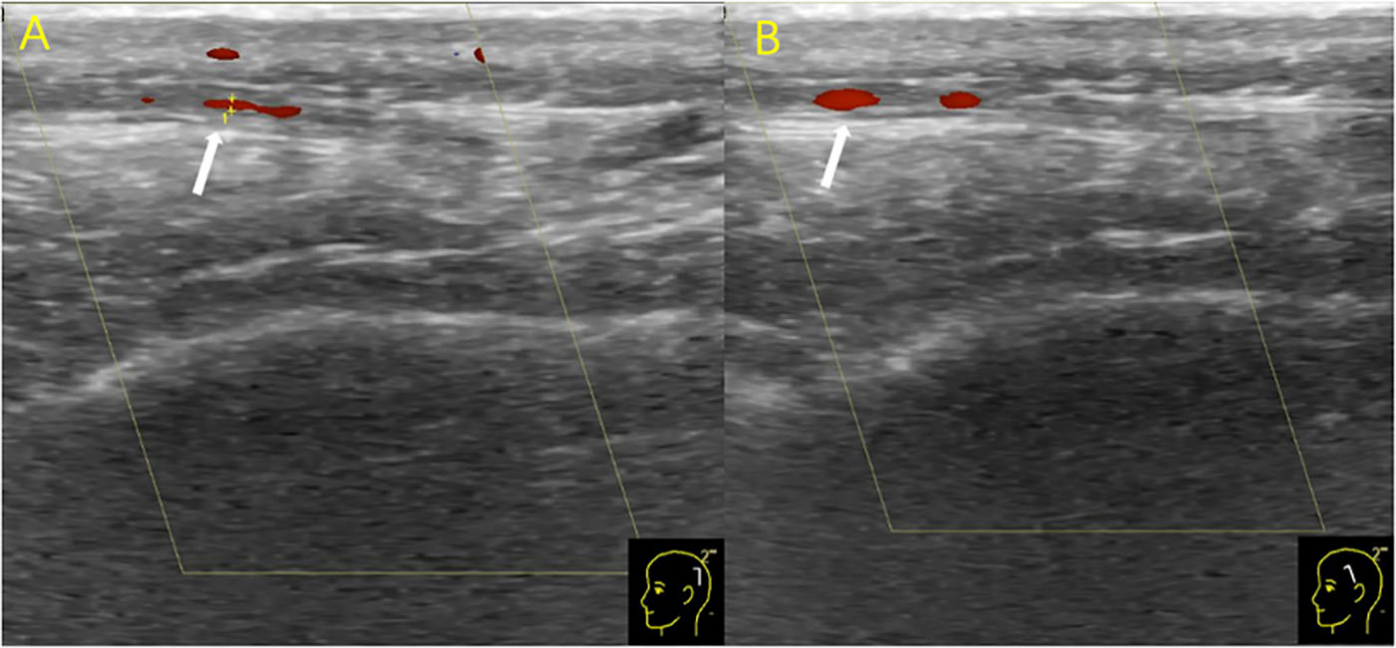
Ultrasound examination revealed the parietal branch (A) and frontal branch (B) of the left superficial temporal artery.
